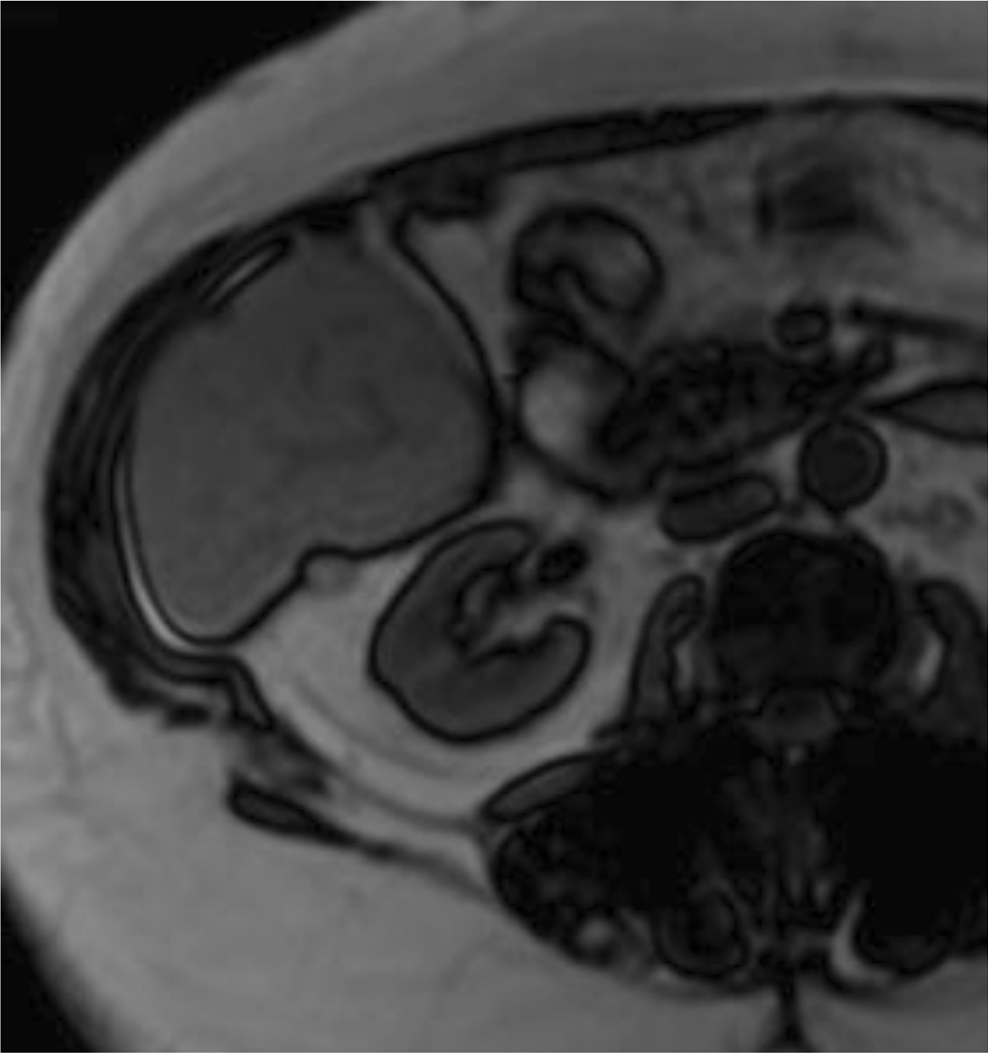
Opposed-phase T1-weighted image demonstrating ‘india ink’ artefact between the pseudolipoma and the liver, but not between the pseudolipoma and adjacent fat in Morrison’s pouch
